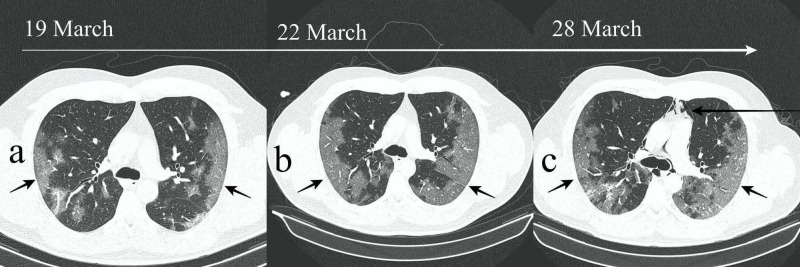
(a-c) Axial HRCT images demonstrating progressive and peripheral predominant ground-glass opacities consistent with COVID-19 (short arrows). Spontaneous pneumomediastinum developed during the second week (long arrow). HRCT, high-resolution CT
